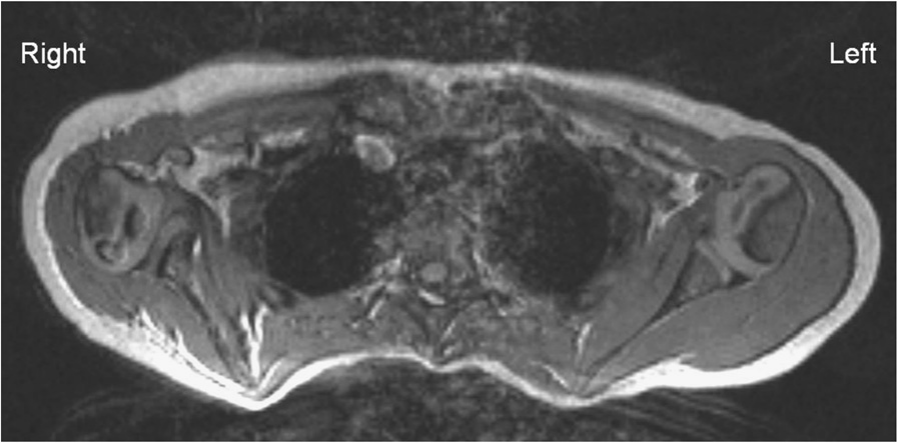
Transversal MRI projection. FISP acquisition MRI in axial plane showing affected and normal contralateral shoulder. In the affected right shoulder there is a convex glenoid form (Birch type 2) and humeral head subluxation. The contralateral left shoulder is normal.
